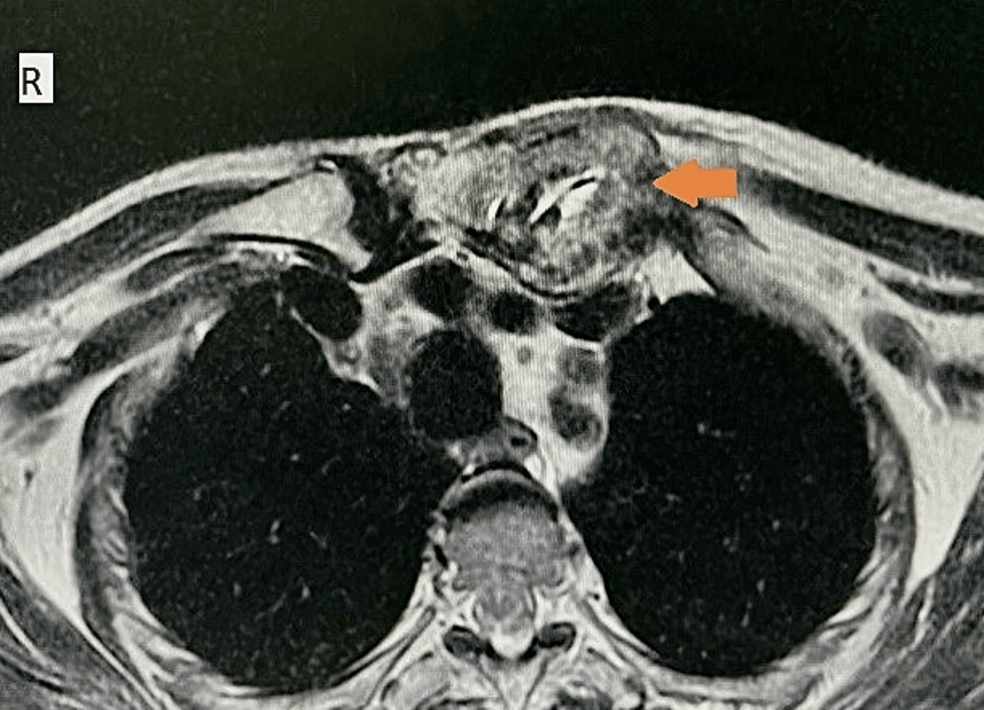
On the axial T2-weighted image, the lesion appears heterogeneous and predominantly hyperintense (arrow).
